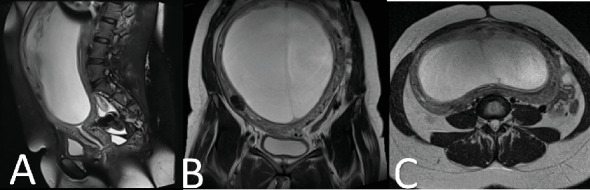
Multiplanar follow-up MRI on the pelvis in the (A) sagittal, (B) coronal, and (C) axial showing a large well-defined T2 hyperintense and T1 hypointense mass in the anterior wall of the uterine cavity, posteriorly effacing the endometrial cavity. There are additional multiple small different-sized T2 hypointense masses located within the myometrium of the uterus.
